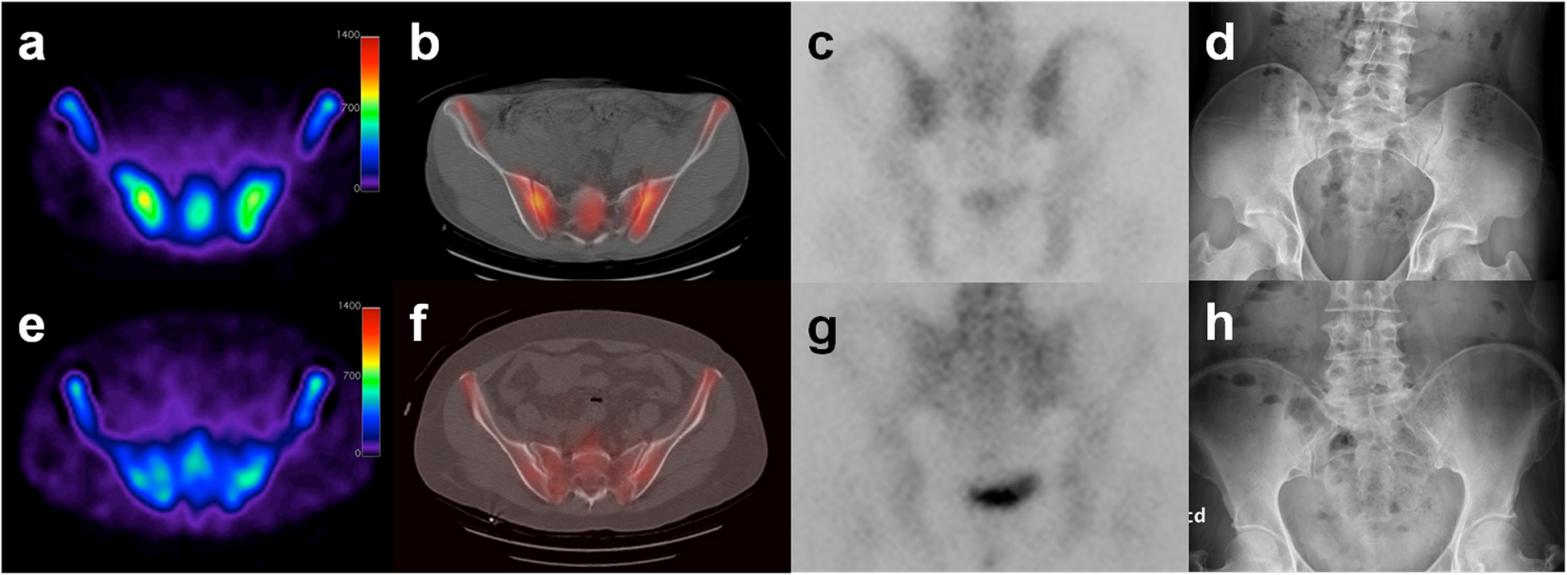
An example of an early axial spondyloarthritis (SpA) patient (a–d). Transaxial images of bone SPECT (a) and SPECT/CT (b) demonstrated uptake in SI joints with high SIS ratios of 1.86 (right) and 1.62 (left). The posterior image of bone scintigraphy (c) showed normal SIS ratios of 1.11 (right) and 1.09 (left). The anterior image of plain radiography (d) showed SI joint grades of 1 (right) and 0 (left). An example of a control subject (e–h). Transaxial images of bone SPECT (e) and SPECT/CT (f) showed comparable SIS ratios of 1.04 (right), and 1.22 (left). The posterior image of bone scintigraphy (g) showed normal SIS ratios of 1.09 (right) and 0.91 (left). The anterior image of plain radiography (h) showed an SI joint grade of 0 (both sides).
